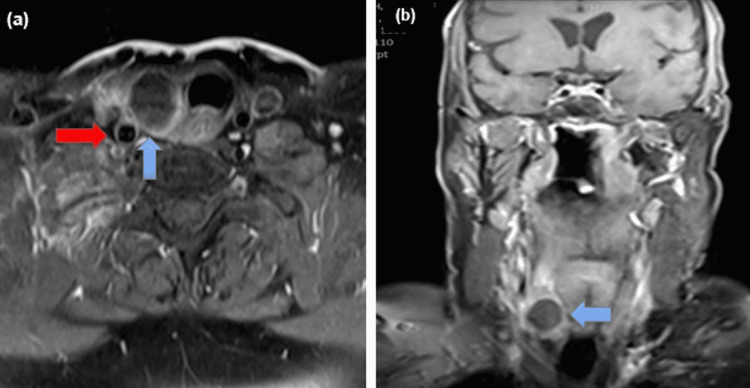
T1 MRI post-gadolinium Transverse (a) and coronal (b) sections demonstrating a right thyroid mass (blue arrow) with internal haemorrhage and laterally focal extra-capsular extension, closely applied to the right common carotid artery to a third of its circumference (red arrow).
